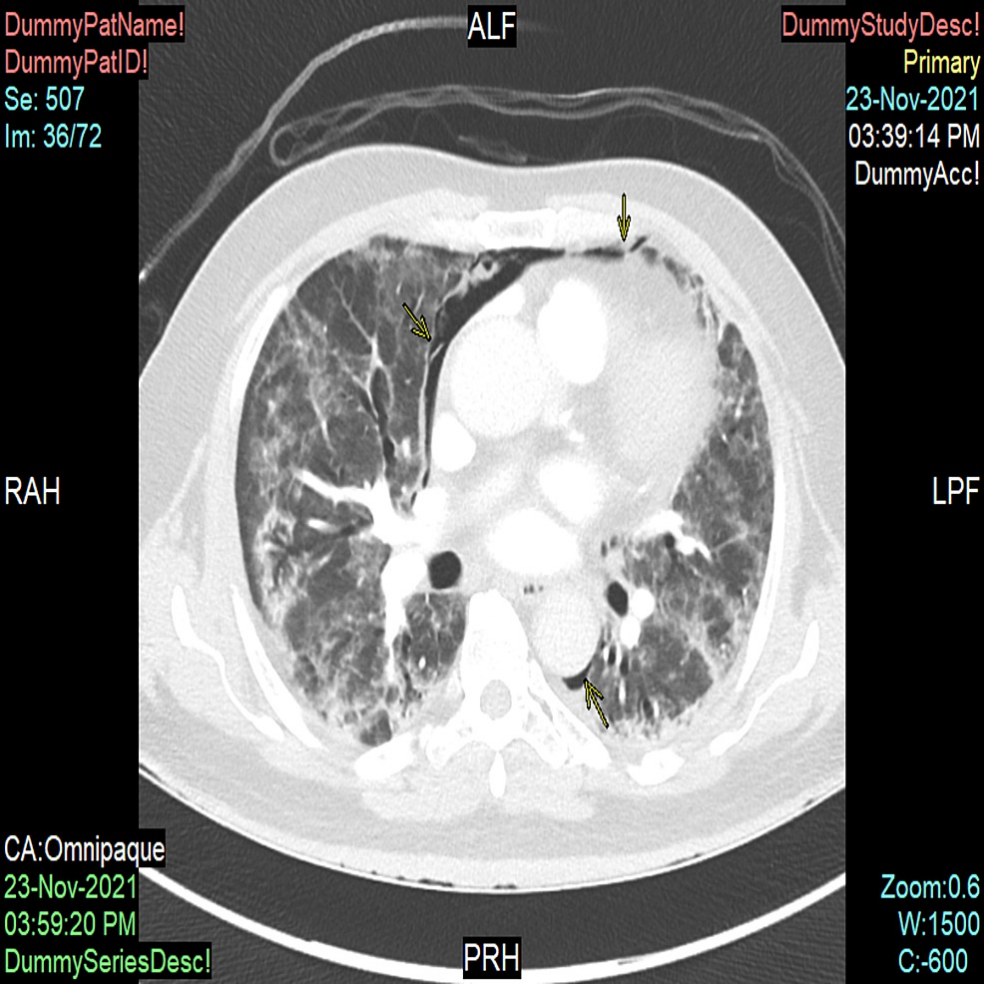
Computed tomography scan in axial view of the patient demonstrating pneumomediastinum (yellow arrows indicating the layering of air alongside pulmonary vasculature)
